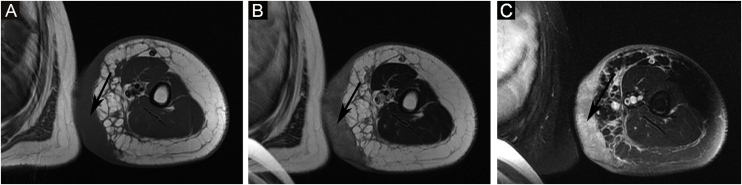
MRI showed nodules distributed on the surface of the skin and subcutaneous tissue; it appeared long T1 and long T2 signals. (A) AXI SE T1. (B) AX T2 FSE. (C) AX fs T2 FSE.
